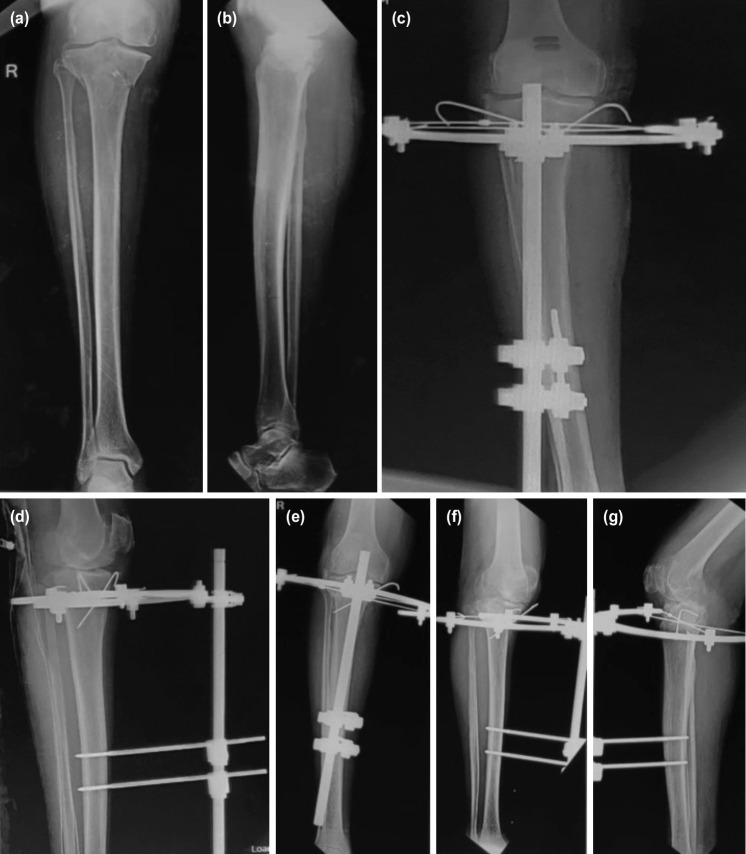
(a,b) Showing the pre-operative radiograph. (c) Showing the immediate post-operative radiograph AP view. (d) Showing the immediate post-operative lateral radiograph. (e,f,g) Showing complete union at the fracture site at three months follow-up.
